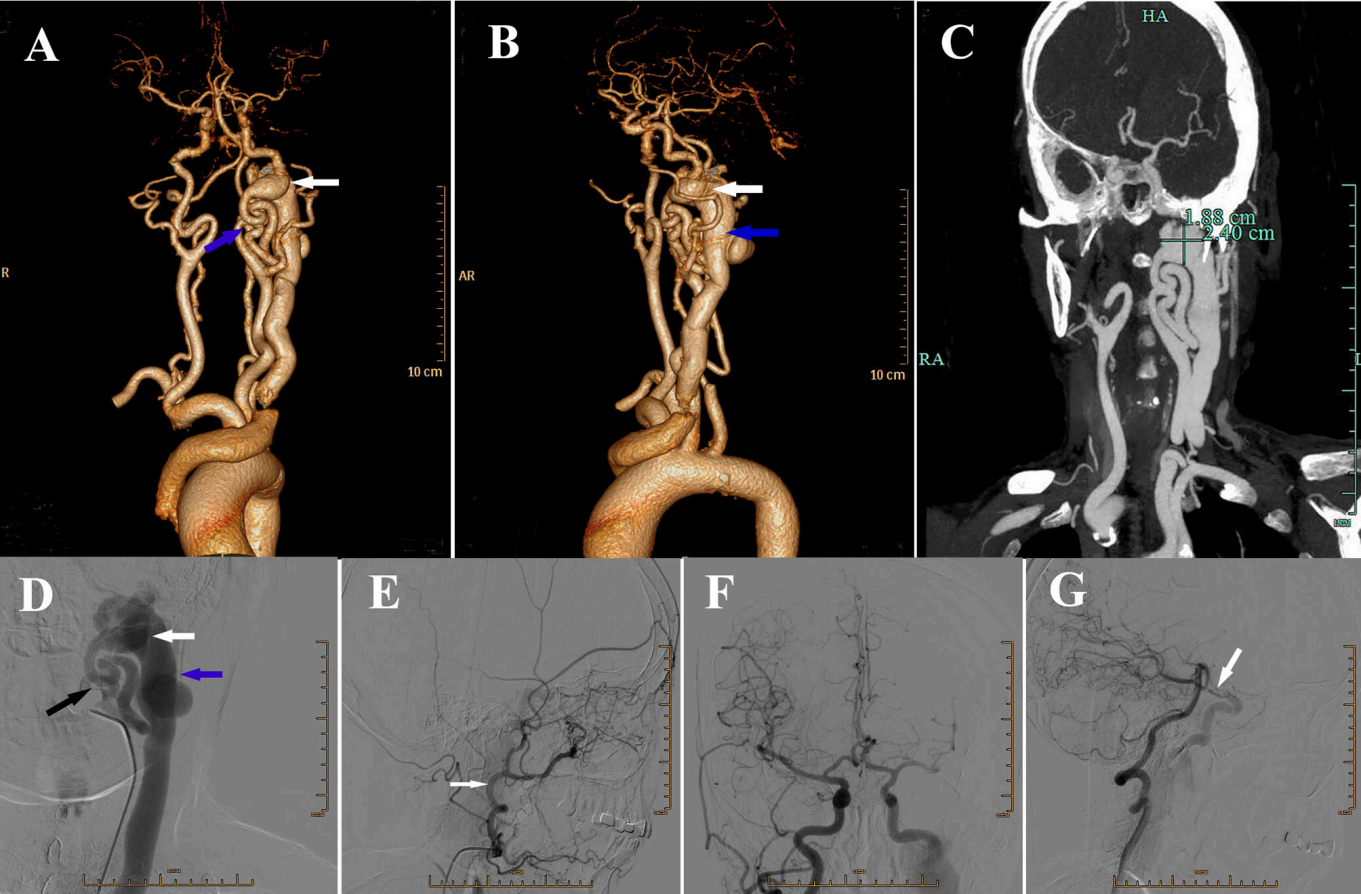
(A) Anteroposterior view of three-dimensional volume rendering of computed tomography angiography (CTA) showing a fistula (white arrow) located at the lever of craniocervical junction, with severe vascular distortion (blue arrow) at the beginning of left internal carotid artery (ICA). (B) Lateral view of three-dimensional volume rendering of CTA showing the dilatation of internal jugular vein (blue arrow). White arrow: the fistula. (C) CTA showing the size of fistula. (D) Digital subtraction angiography (DSA) showing the fistula (white arrow), dilatation of internal jugular vein (blue arrow) and the absence of blood flow from left ICA (black arrow) into the brain. (E) DSA of left external carotid artery (ECA) (white arrow) showing no obvious abnormality. (F) DSA showing right ICA supplying the left anterior cerebral artery and middle cerebral artery via the anterior communicating artery. (G) DSA showing vertebral basilar artery supplying contralateral cerebral via left posterior communicating artery (white arrow).
